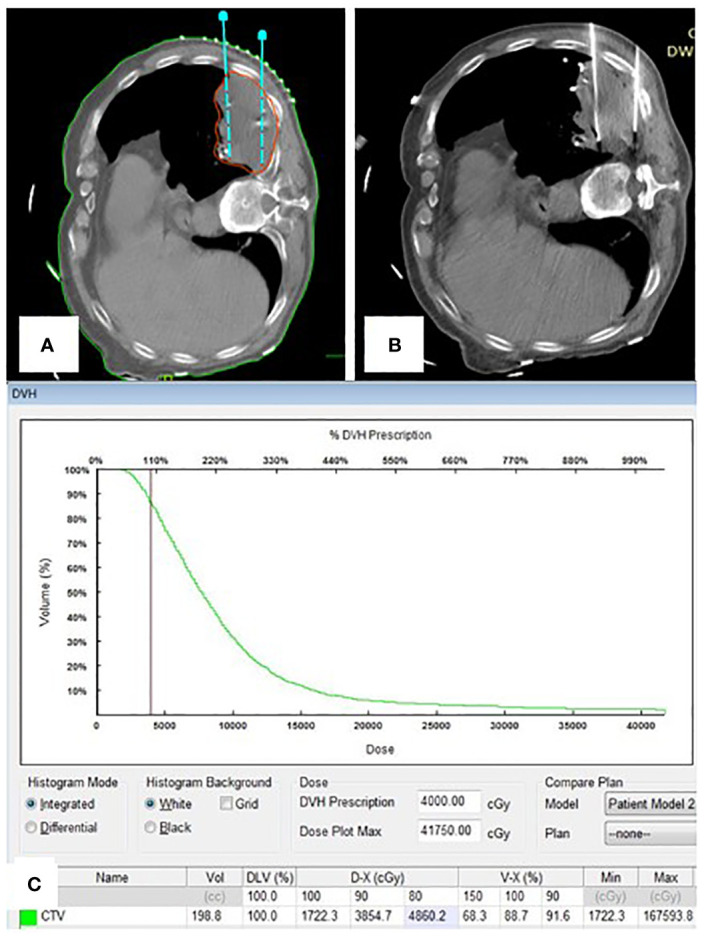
(A) Portion of pre-plan images; (B) Portion of real-time CT guided 125I seed implantation; (C) Dose-volume histogram of 125I seeds postoperative.
